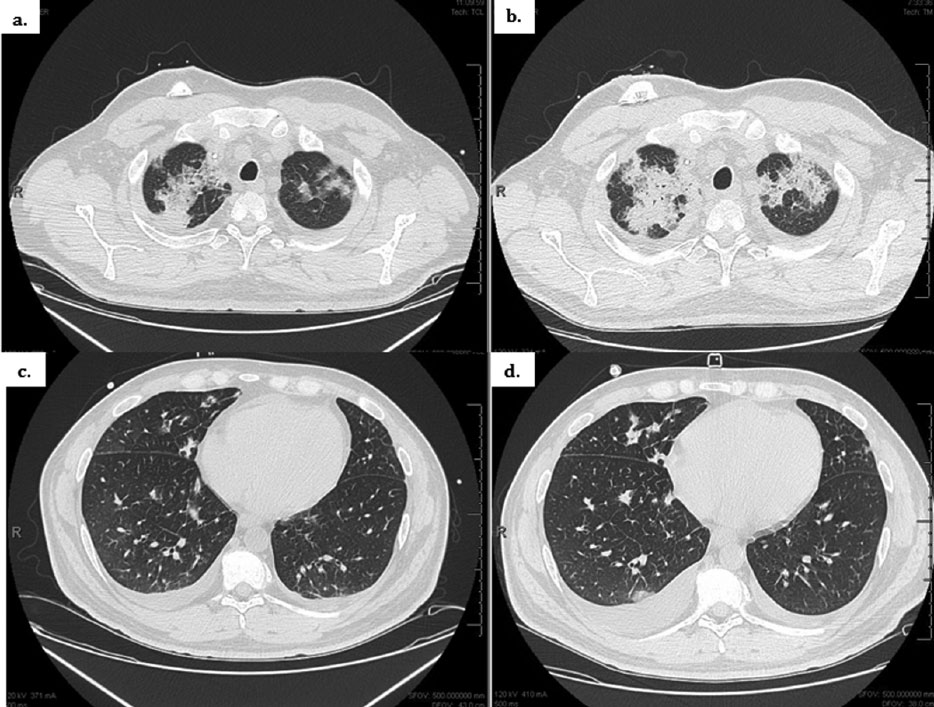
Comparative chest CTs. Admission CT scan (left; [a] upper lung zone, [c] lower lung zones). CT scan 9 days later (right; [b] upper lung zone, [d] lower lung zone) with worsening bilateral patchy and confluent airspace opacities.
